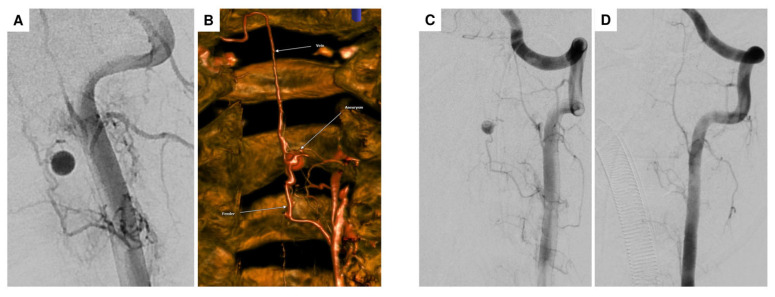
DSA in the anteroposterior plane showing the intramedullary arterial aneurysm at the C2 level, associated with an arteriovenous (AV) shunt originating from an arterial feeder of the left vertebral artery at the C3/4 level, consistent with a perimedullary AV fistula (A). Flat-panel CT angiography with volume rendering technique (VRT) reconstruction clearly shows the AV fistula morphology, including the arterial feeder (bottom arrow), the intramedullary aneurysm (middle arrow), and the draining shunt vein (top arrow) (B). Compared to preoperative DSA (C), no residual aneurysm or shunt vein was observed postoperatively, confirming complete occlusion of the AV fistula (D).
